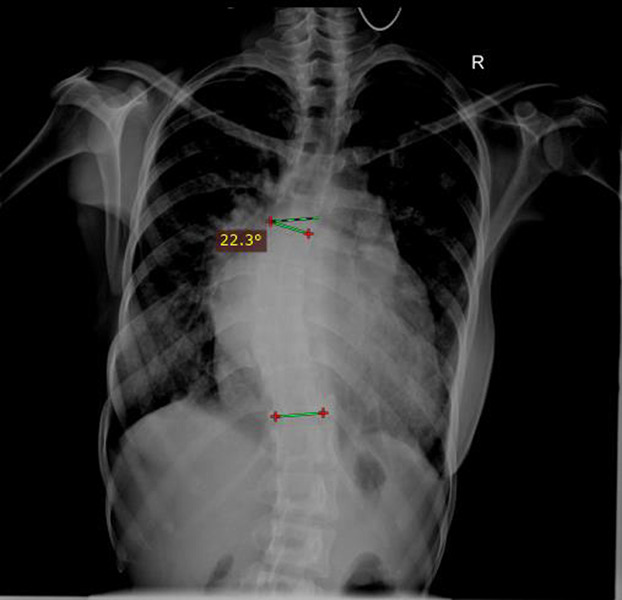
A plain chest radiograph of an 18-year-old girl with Cobb angle measurement of 22.3^0^
